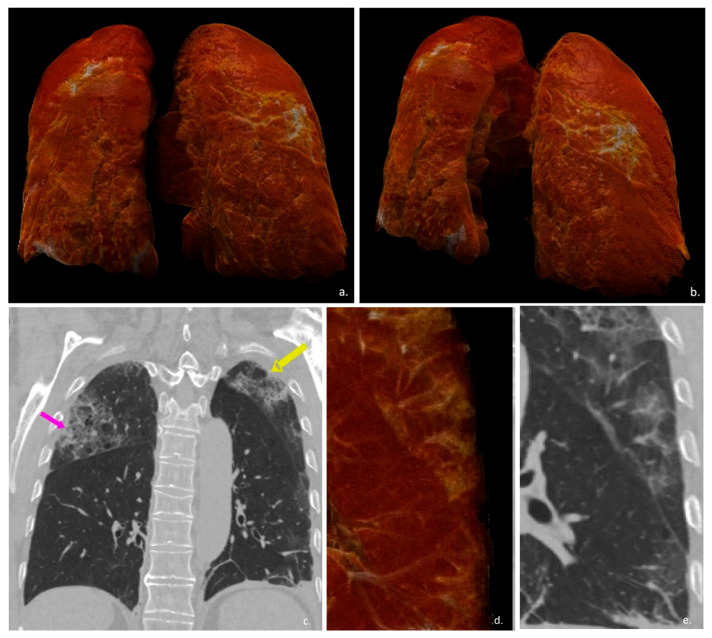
Automatic lung volume detection. Two different views from a volume-rendered reconstruction were obtained from the automatic detection of lung tissue (img (a,b)). Pink and yellow arrows in img (c) highlight also high-density lesion within lung tissue, in a coronal view. In images (d,e), magnified, volume-rendered reconstruction and coronal CT scan view (respectively) enhance the high capability of CT and quantitative reconstruction to detect also millimetric lesion.
